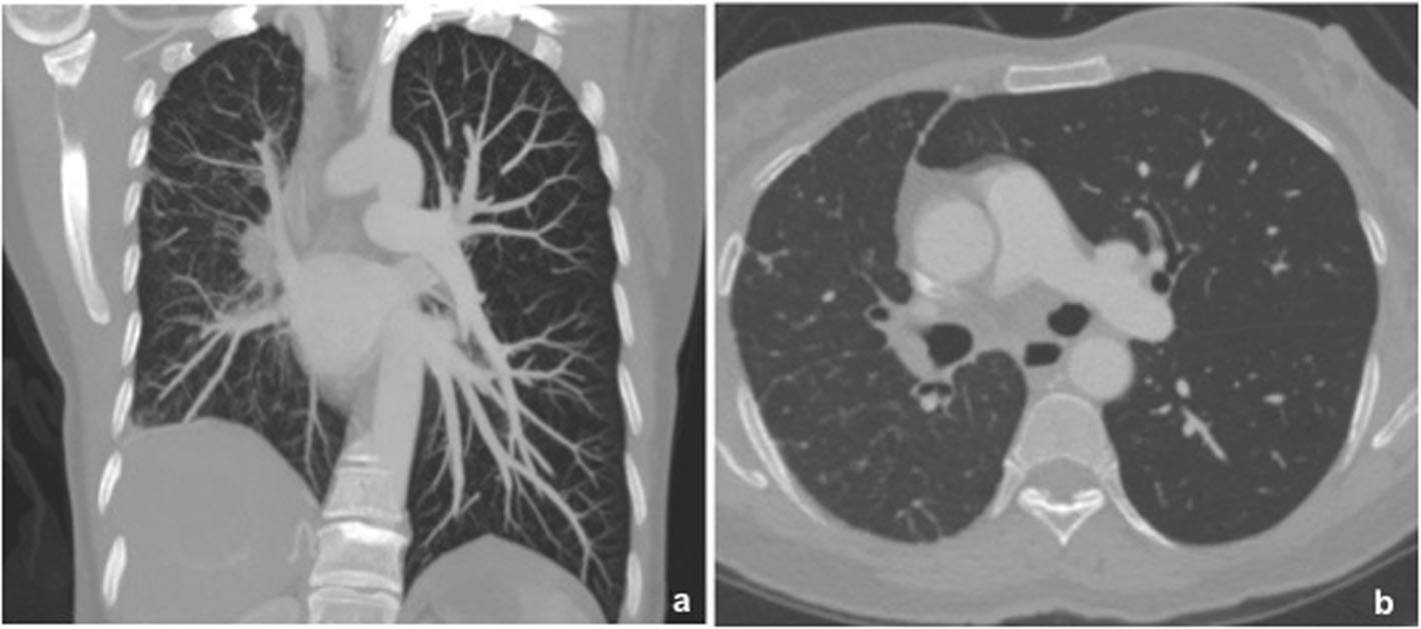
Chest CT of a 48-year-old woman demonstrated right pulmonary artery agenesis. Coronary CT reconstructed image showed smaller hemithorax, elevation of the hemidiaphragm and paucity of lung vascular markings on the right lung suggesting right pulmonary artery agenesis (a). Axial contrast-enhanced CT revealed the absence of right pulmonary artery within 2 cm of its expected origin from the main pulmonary artery (b)
